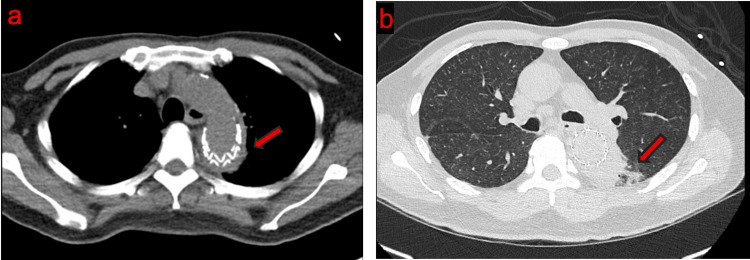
Periaortic fluid and/or aortic wall thickening with adjacent pulmonary consolidation favors the diagnosis of TA. (a) Axial contrast-enhanced CT at 20 weeks demonstrating an endovascular stent graft fully covering the previously identified PAU, with associated periaortic fluid or aortic wall thickening in the setting of minimal underlying atherosclerotic disease (red arrow). (b) Lung window demonstrating persistent consolidation in the left lung adjacent to the descending thoracic aorta (red arrow).
